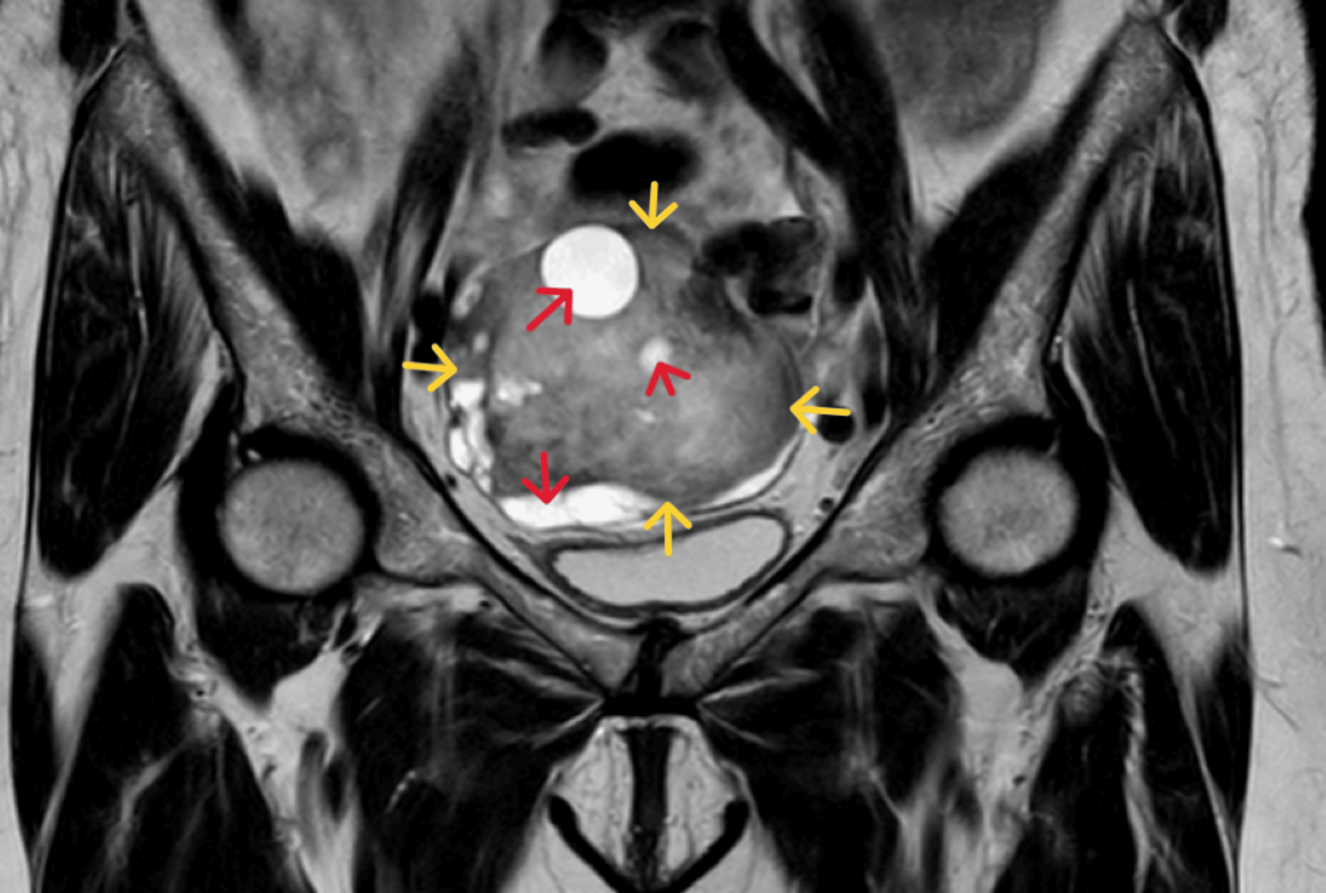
MRI of the ovarian thecoma The lesion shows low-intensity signal in both T1 and T2 sequences (yellow arrows) with signs of cystic degeneration (red arrows)
